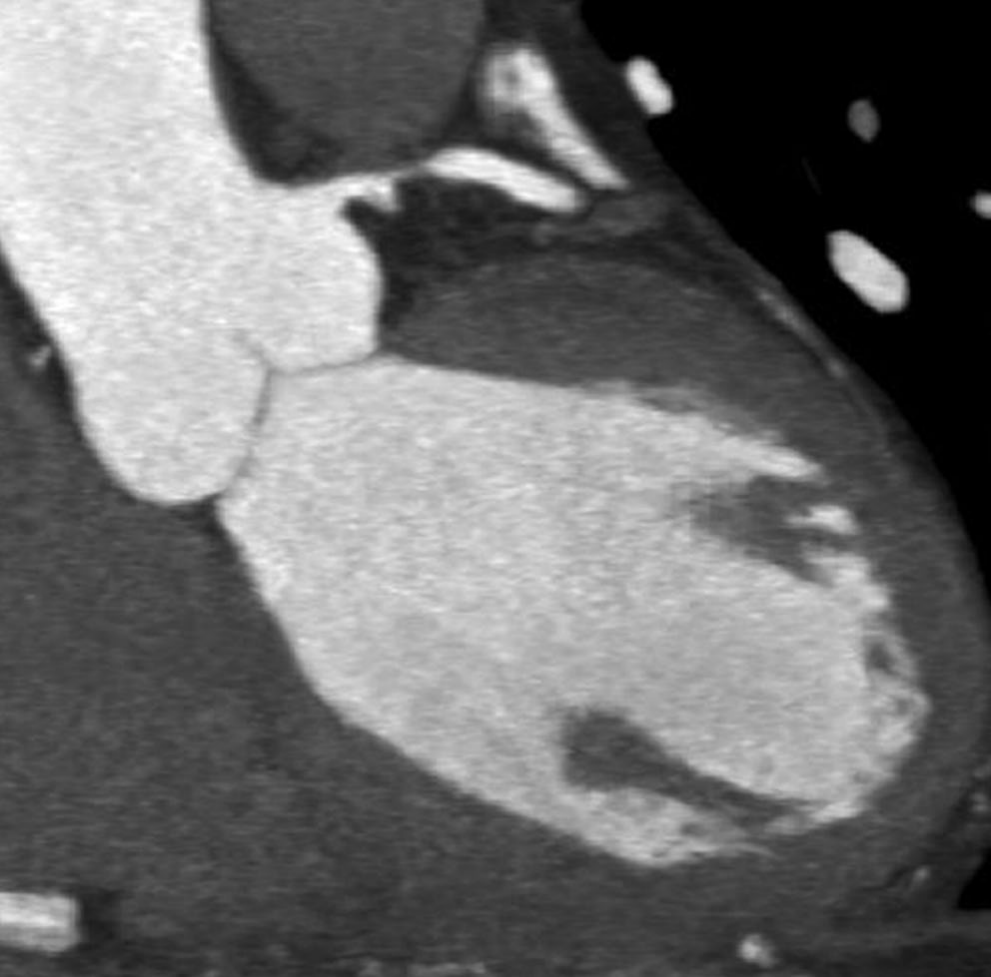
Thick maximum intensity projection coronary CT angiography image
